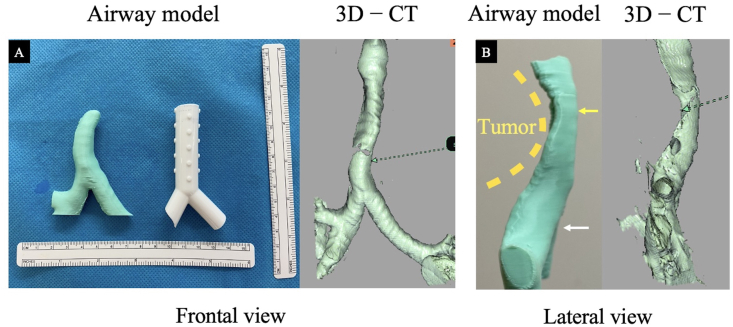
Comparison of the airway model and 3-dimensional (3D)-computed tomography (CT) images. (A) Comparison of the airway model, trimmed stent, and 3D-CT image in the frontal view showing that the airway model has almost the same shape as the 3D-CT image and that the silicone stent can be cut precisely to allow proper opening of the silicone stent into the right upper lobe. (B) The airway model in the lateral view is comparable to the 3D-CT image, and the distance between the tracheal bifurcation (white arrow) and the malignant airway stenosis site (yellow arrow) is easy to understand.
